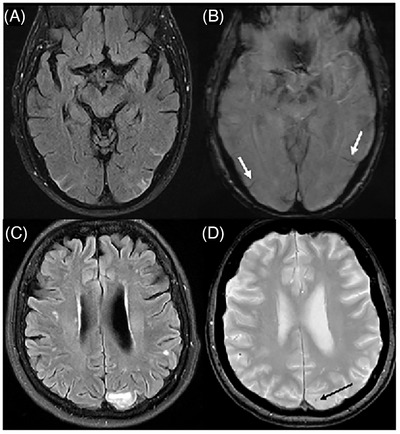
Examples of ARIA‐E and ARIA‐H. Patient 1 (A, B) with moderate ARIA‐E (effusion, edema) and moderate ARIA‐H (siderosis). (A) FLAIR MRI demonstrates hyperintense bilateral occipital and left posterior temporal sulcal effusions and left occipital vasogenic edema. (B) Corresponding susceptibility‐weighted imaging (SWI) has bilateral linear hypointensities, consistent with superficial siderosis (white arrows). The patient had mild symptoms (reported transient blurry vision but denied headache, dizziness, or other symptoms). Dosing was suspended, and the patient was scheduled for a 1‐month follow‐up MRI. Patient 2 (C, D) with mild ARIA‐E and mild ARIA‐H. (C) FLAIR demonstrates hypertense signal and mild mass effect in the superior left occipital measuring up to 2 cm. (D) Gradient echo (GRE) imaging shows one new microhemorrhage in the same region (black arrow). The patient was asymptomatic. Dosing was suspended, and the ARIA‐E resolved on the 1‐month follow‐up MRI. ARIA, amyloid‐related imaging abnormalities; ARIA‐E, ARIA manifesting as vasogenic edema; ARIA‐H, ARIA manifesting as hemorrhagic lesions; FLAIR, fluid‐attenuated inversion recovery; MRI, magnetic resonance imaging.
